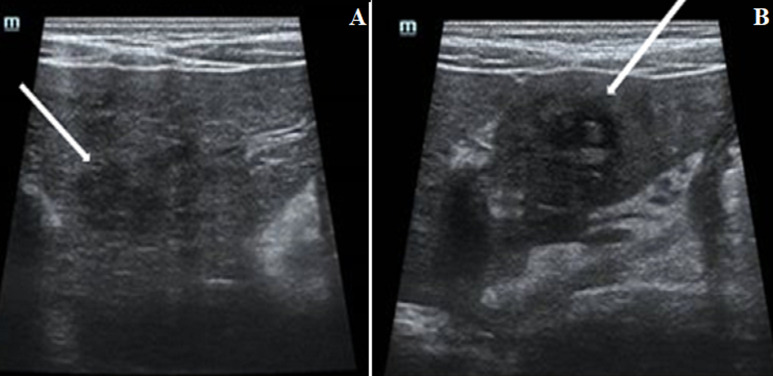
ultrasound images of the liver showing poorly defined heterogeneous hypoechoic areas of variable shape (white arrow), visible in segments IV (A) and III (B) of the liver
